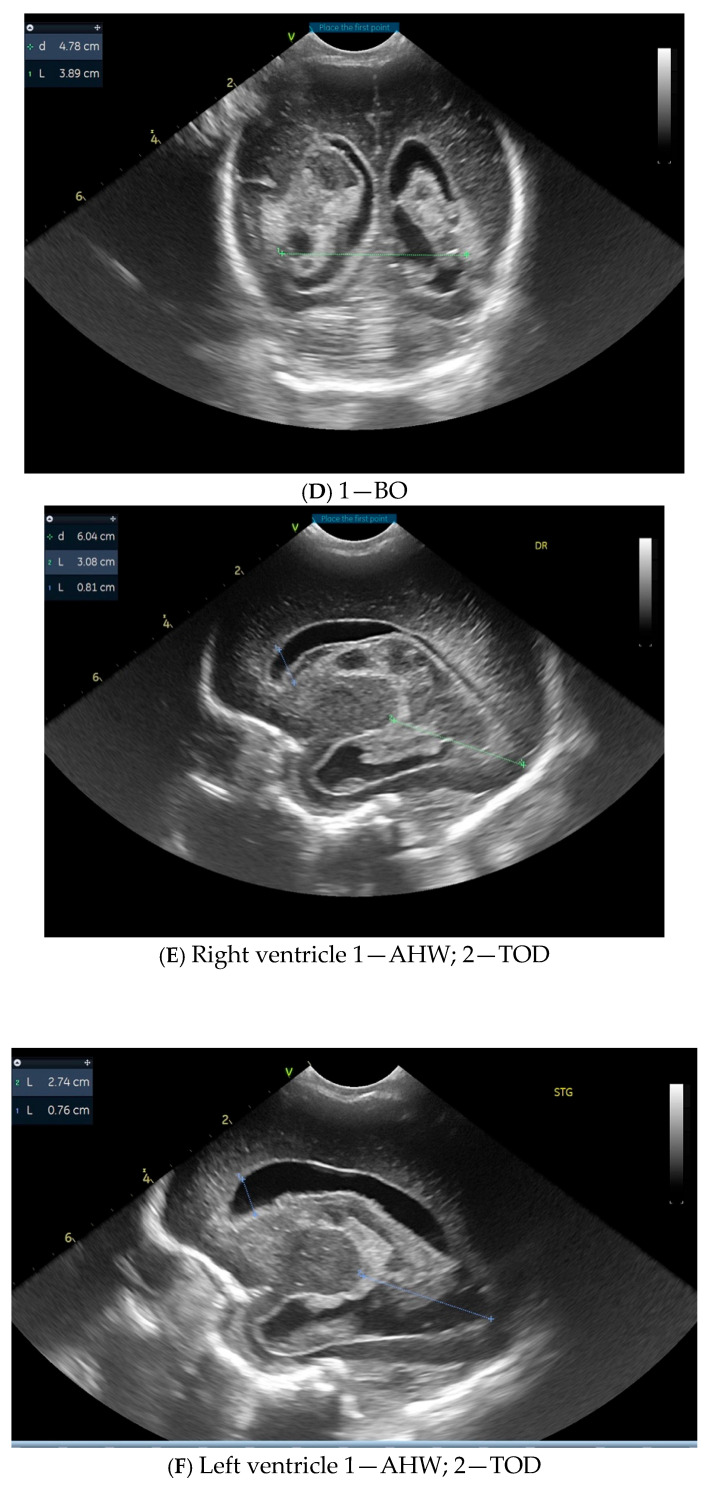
Images from cranial ultrasound of a 26 weeks of gestation preterm with different measurements. (A,B) = Coronal cUS at level of Foramen of Monro, showing extensive echogenic material within the lateral ventricles, consistent with intraventricular hemorrhage. Ventricular dilatation is also noted, reflecting post-hemorrhagic changes. (C,D) = coronal cUS at the level of occipital horn, showing the hemorrhage that extends into the adjacent periventricular white matter, visible as areas of increased echogenicity, indicating parenchymal involvement and venous infarction. (E,F) sagittal cUS with lateral ventricles that are globally enlarged and the intraventricular clot that appears as an inhomogeneous, irregular hyperechoic mass that occupies more than 50% of the ventricular volume. VI = Ventricular Index, AHW = Anterior Horn Width, TOD = Thalamo-Occipital Diameter; BF = bifrontal horn, BP = biparietal, BO =bioccipital horn.
